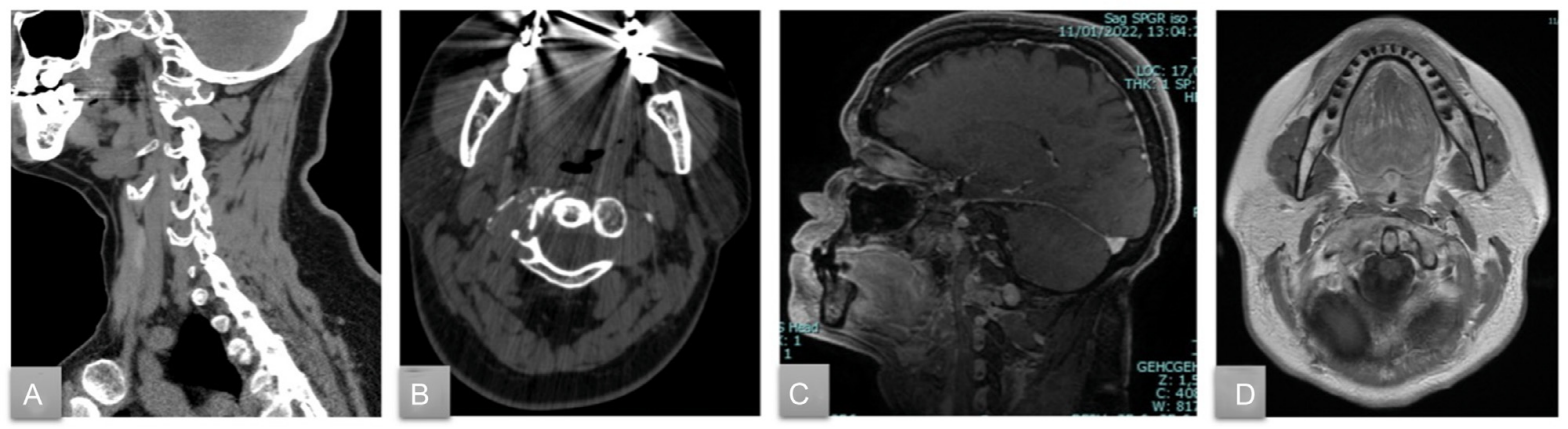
Preprocedural sagittal (A) and axial (B) CBCT scan showing the osteolytic lesion at the C1 vertebra. Preprocedural sagittal SPGR c+ (C) and axial T1 TSE c+ (D) MRI confirming the osteolytic lesion of C1 with heterogeneous postcontrast enhancement.
